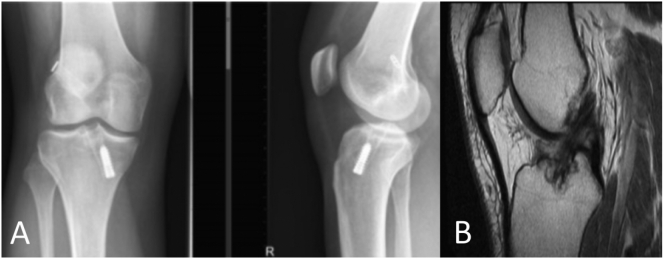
A. Plain X-rays AP and lateral right knee (patient 3). Adequate tunnel positioning. B. Sagittal MRI image of an intact ACL graft (patient 3).
